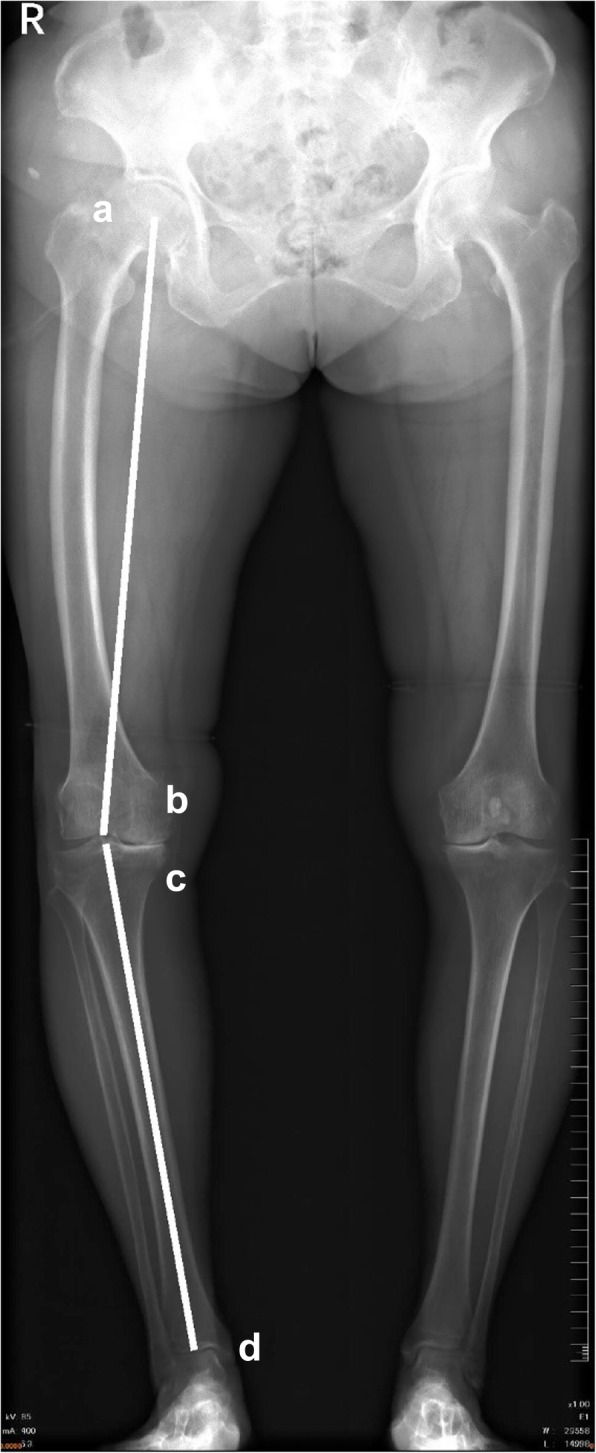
Measurement of knee alignment (hip-knee-ankle angle) based on full-leg standing anteroposterior radiographs. a The center of the femoral head. b The center of the femoral condyles. c The center of the tibial plateau. d The center of the superior surface of the talus
